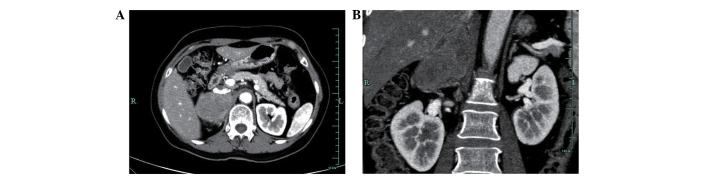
(A) Axial contrast-enhanced computed tomography (CT) scan showing the right adrenal tumor. (B) CT coronal section showing the right adrenal tumor.
